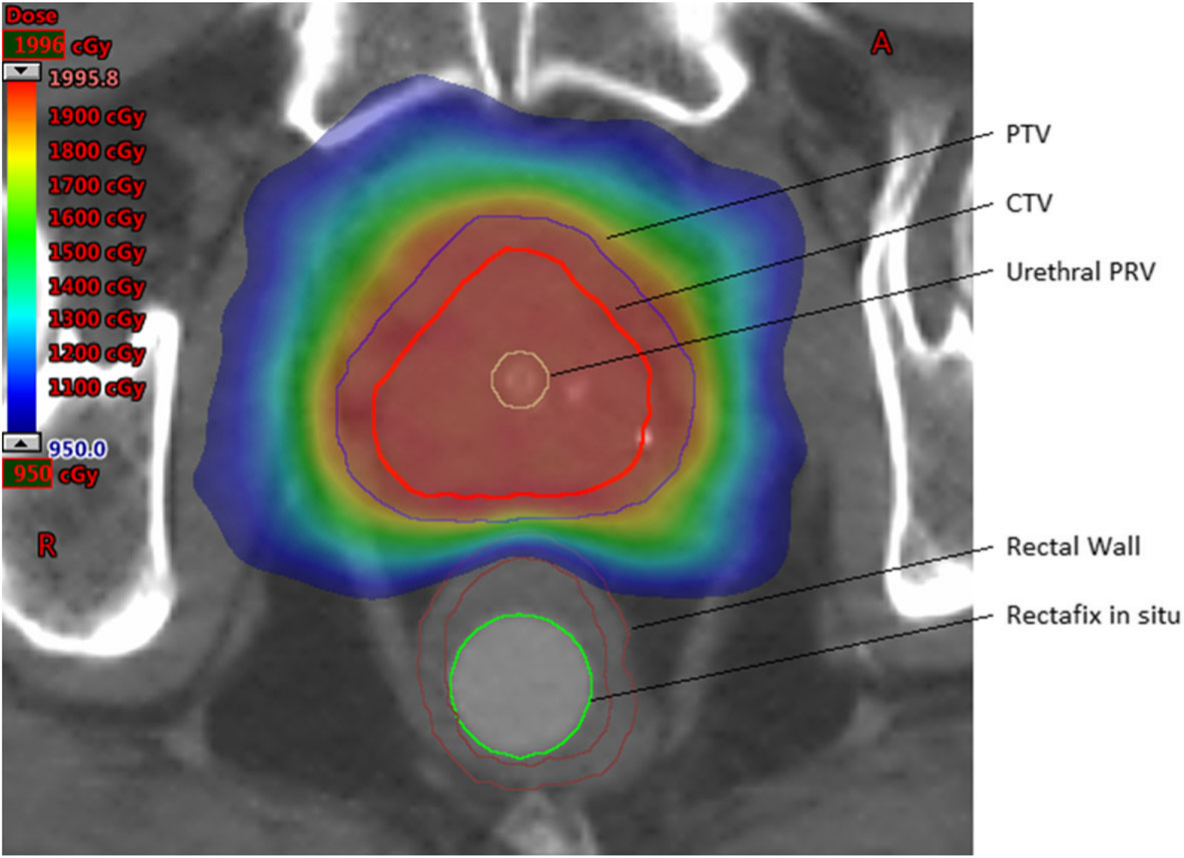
Transverse CT view of example dose distribution adhering to planning constraints with Rectafix™ displacing rectum posteriorly
